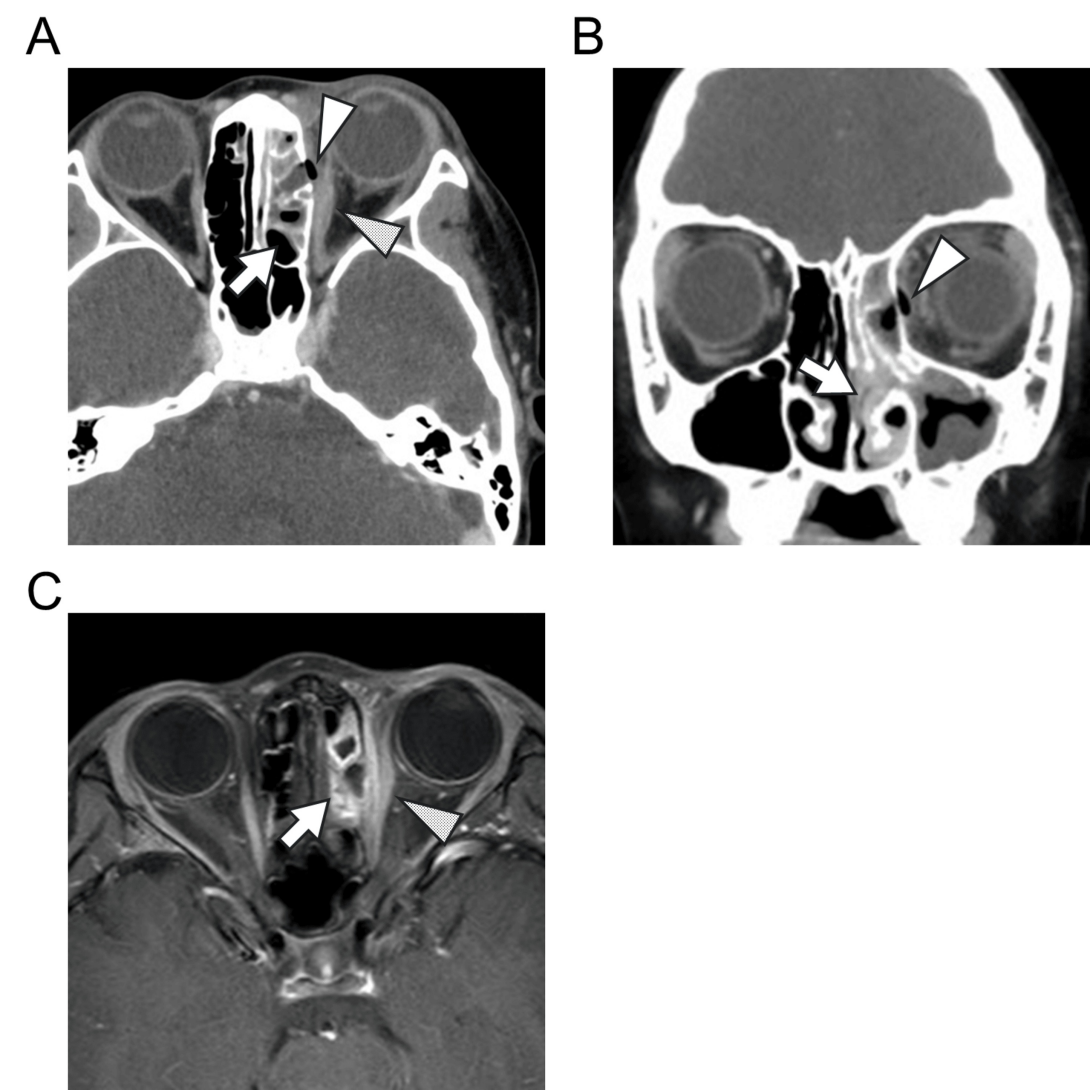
Imaging studies of the patient showing left orbital cellulitis (A) Axial contrast-enhanced CT of the orbit on admission. Cellulitis of the eyelid extended to the cheek. Sinusitis (arrow), air in the orbit (white arrowhead), and mild thickening of the medial rectus muscle (dotted arrowhead) are noted on the left side. (B) Coronal contrast-enhanced CT of the orbit on admission. Sinusitis (arrow) and air in the orbit (white arrowhead) are noted on the left side. (C) Contrast-enhanced T1-weighted MRI of the orbit (axial view). Residual left sinusitis (arrow) is observed. The contrast effect is noted in the left medial rectus muscle (dotted arrowhead). No abscess is observed in the orbit.
